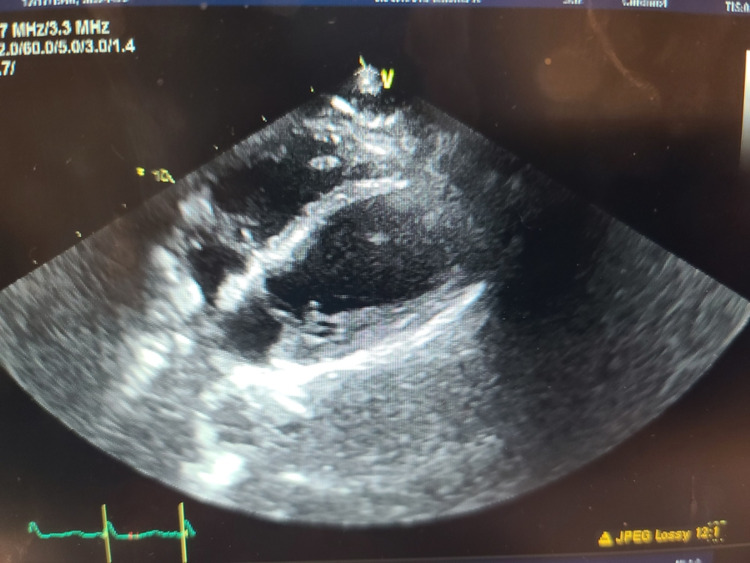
Echocardiogram findings consistent with low ejection fraction and Takotsubo cardiomyopathy
